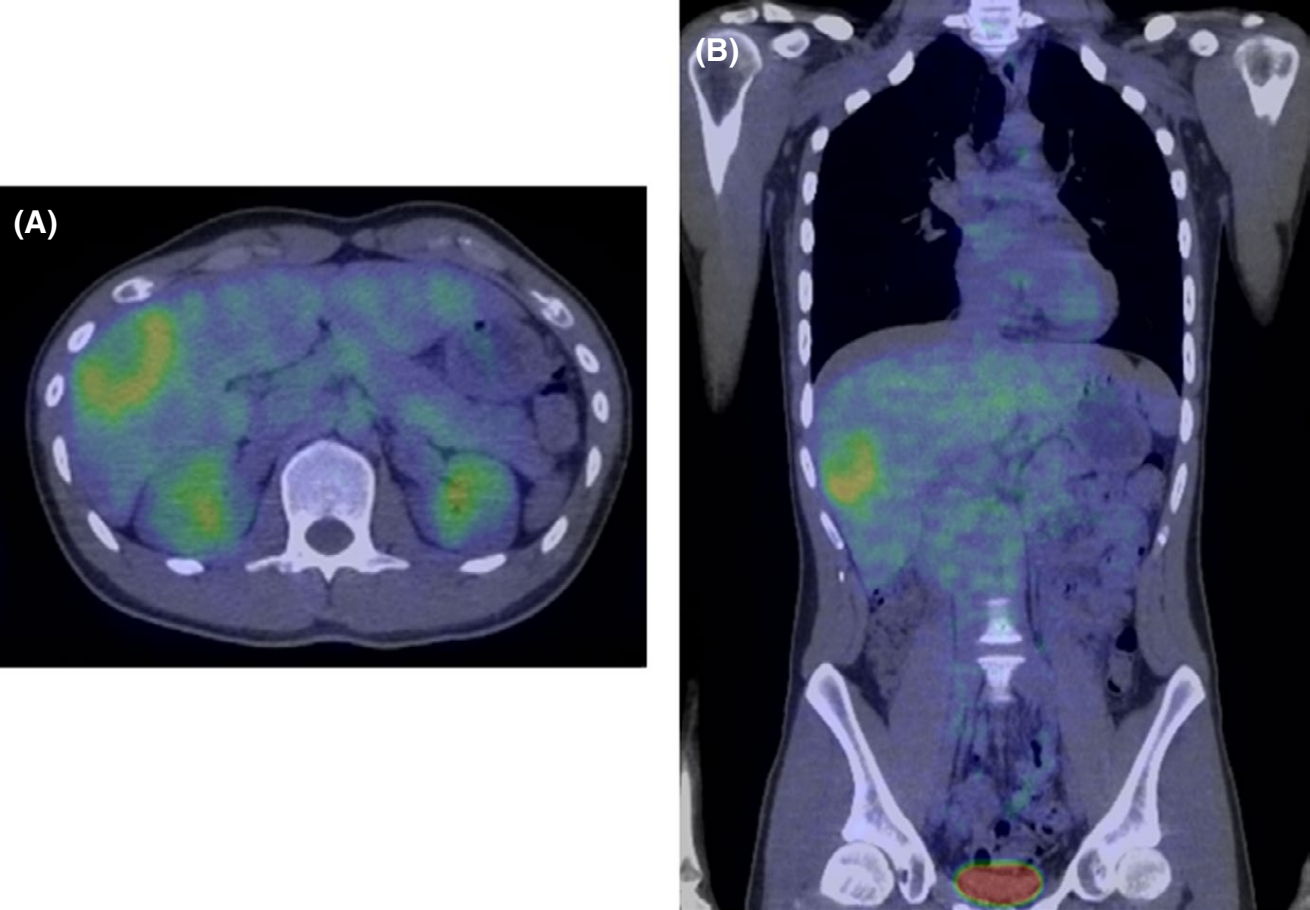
A and B, Fluorin‐18 fluorodeoxyglucose positron emission tomography CT revealed that the tumors had a high accumulation, with a maximum standardized uptake value of 4.9, unlike the other organs
